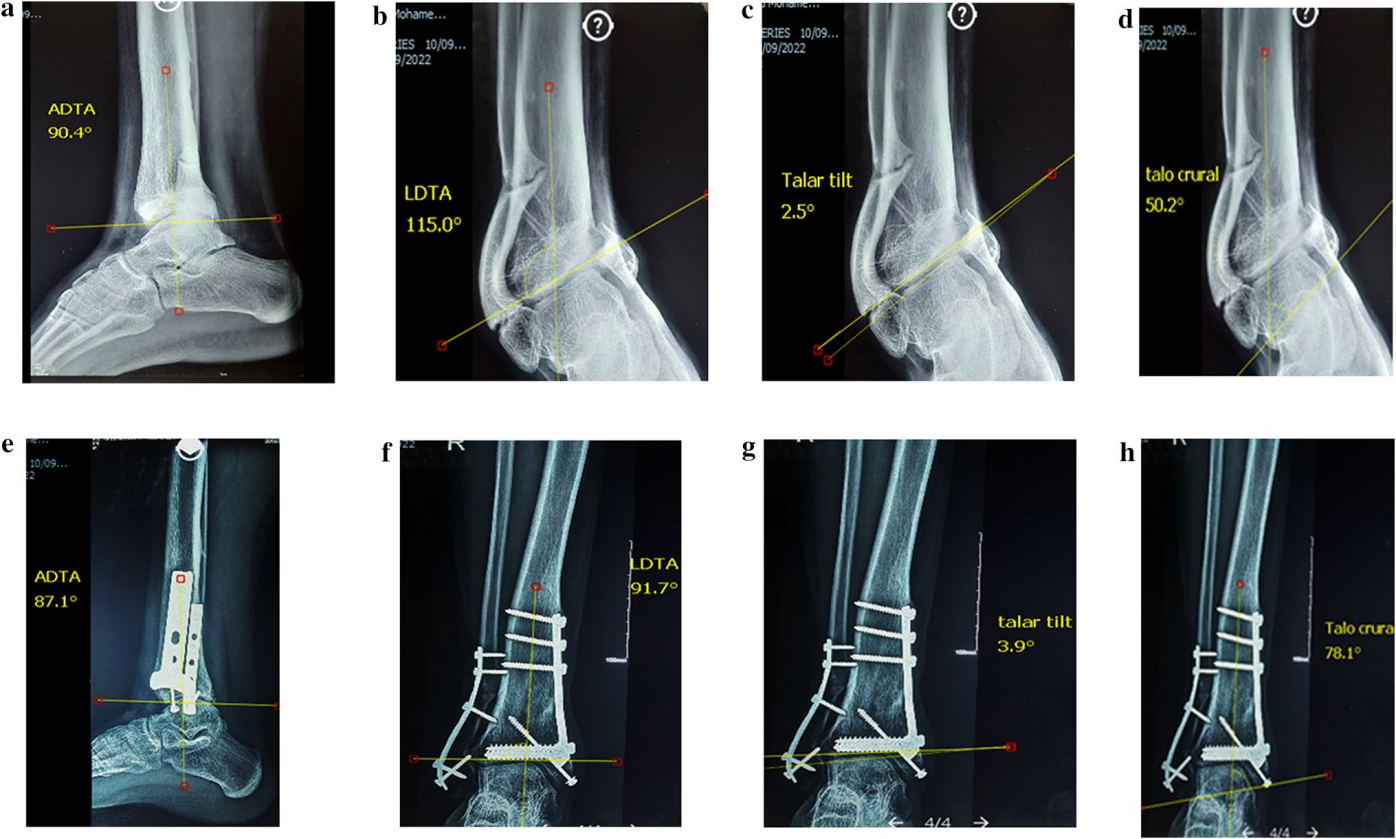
A Male patient 22 years old presented after one year from trauma by post-traumatic right ankle OA with varus deformity and nonunion of distal third fibular fracture and medial malleolus. Medial wedge opening supramalleolar osteotomy with bone graft, fibular plating, and screw fixation of the medial malleolus were done. a–d Preoperative X-rays show degrees of anterior distal tibial angle (ADTA), lateral distal tibial angle (LDTA), talar tilt (TT), and talocrural angle (TCA). e–h X-rays show degrees of ADTA, LDTA, TT, and TCA after 8 weeks with the union of supramalleolar osteotomy, fibular fracture and medial malleolus.
